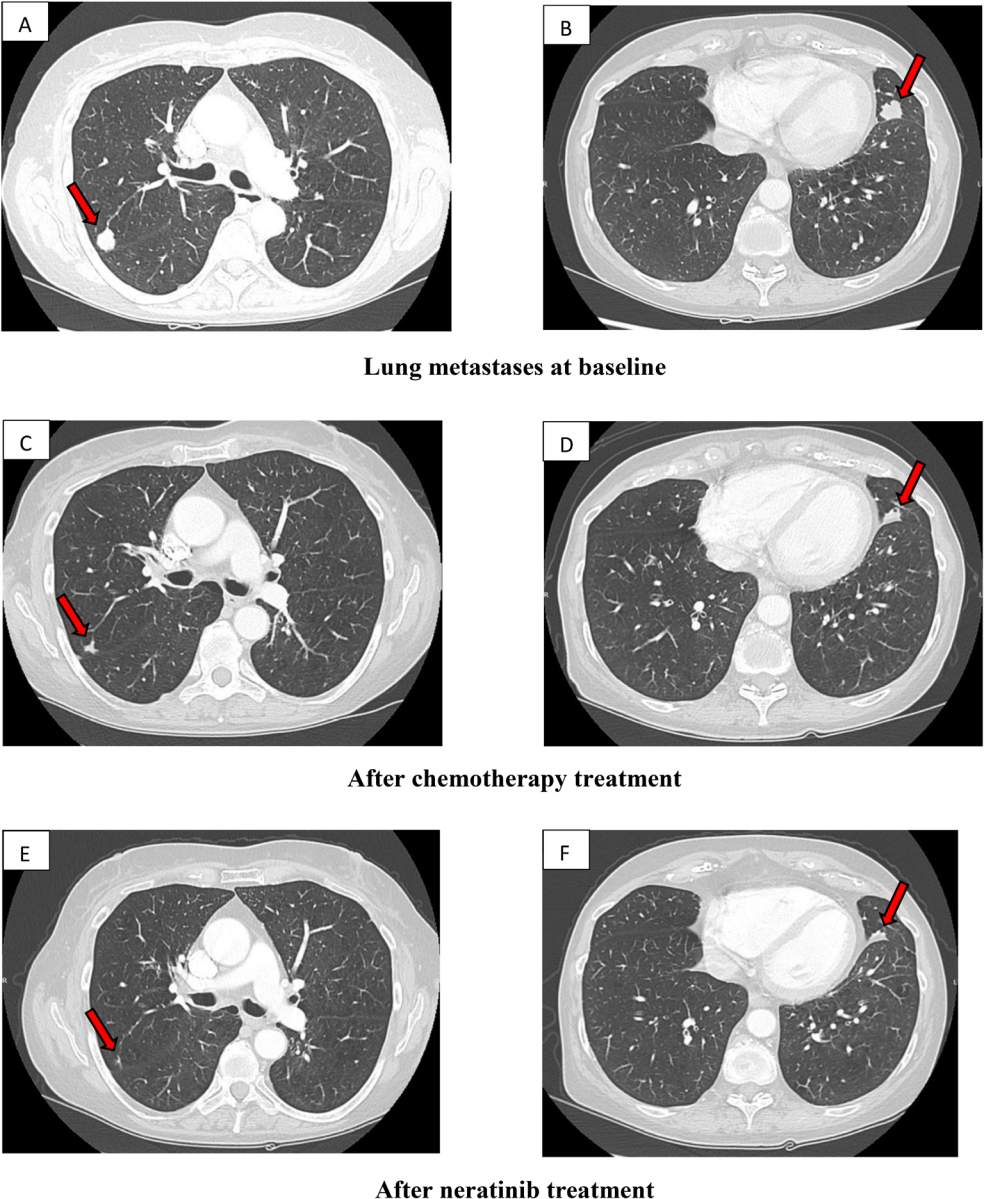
Chest computed tomography images. (A, B) CT scan revealed multiple lung metastases at baseline. (C, D) CT reexamination showed size reduction of lung metastases after chemotherapy treatment. (E, F) CT reexamination showed substantial stability of lung metastases after 3 months of neratinib treatment.
